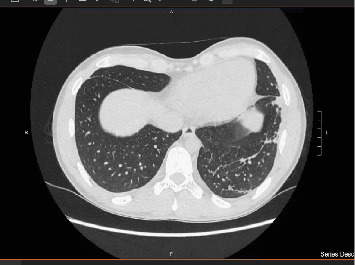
CT thorax at 6-month follow-up.
